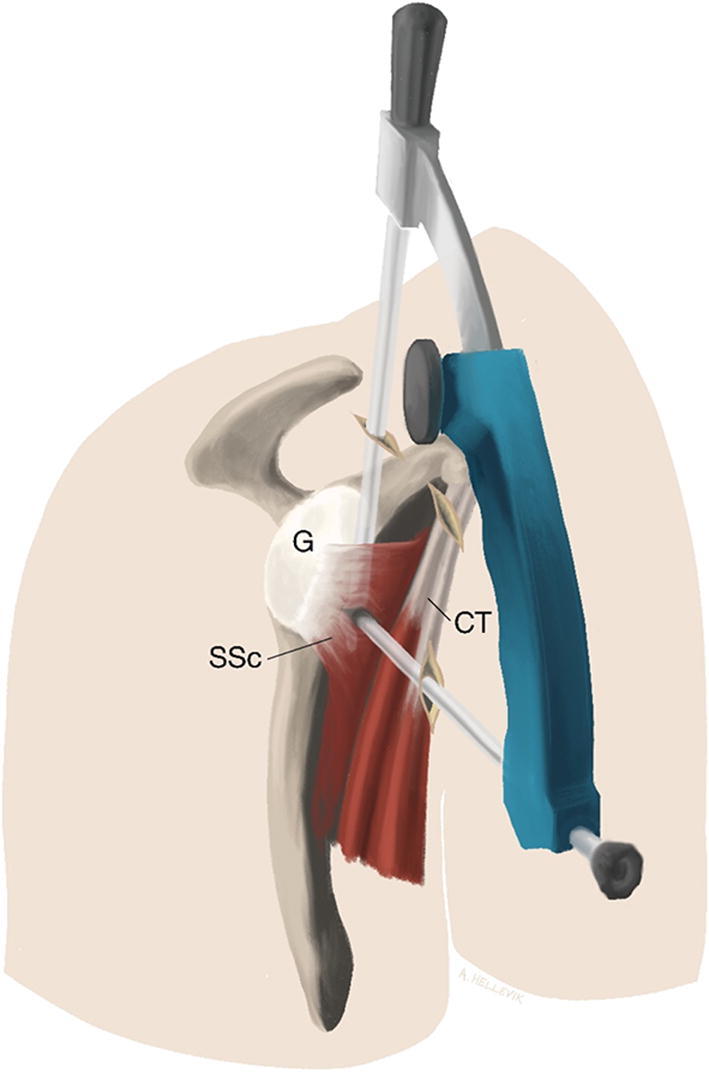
Creating the slit in the subscapular tendon. Subscapular tendon (SSc), conjoined tendon (CT), glenoid (G). The lower anterior portal is created lateral to the conjoined tendon to avoid structures at risk (axillary and musculocutaneous nerves) using an aiming device for AC-joint repair (Arthrex GmbH), switching stick and halfpipe instruments. With help of a switching stick and dilatators, the slit is expanded and a halfpipe is introduced through the lower anterior portal. A banana knife was used to enlarge the slit from medial to lateral
